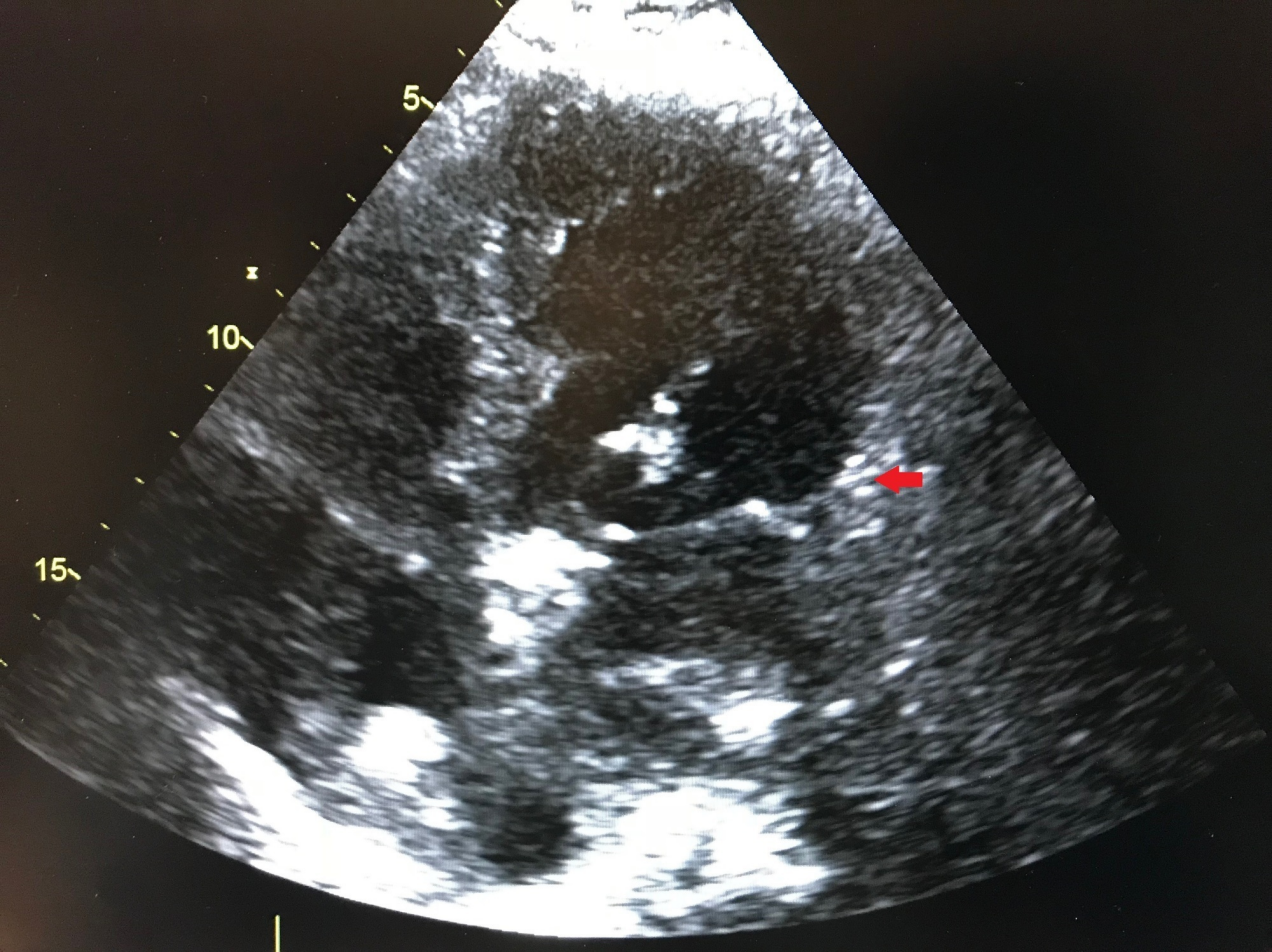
Repeat echocardiogram (apical 2 chamber view) showing mild residual hypokinesis of the left ventricle apex (arrow).
